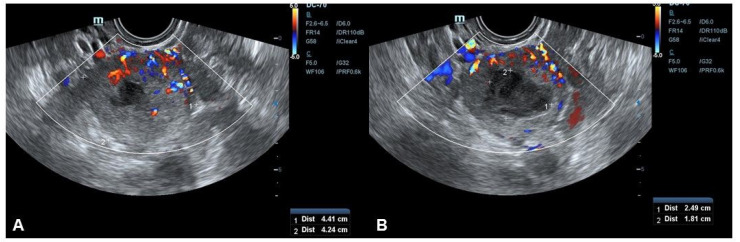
Transvaginal ultrasound showing a subserosal mass 4 cm in size with well-delimited borders and a central hypoechoic portion that was 25 × 18 mm (A,B). Color Doppler showed a tumor with a rich, irregular, central vascular network (A) (color score 3).
